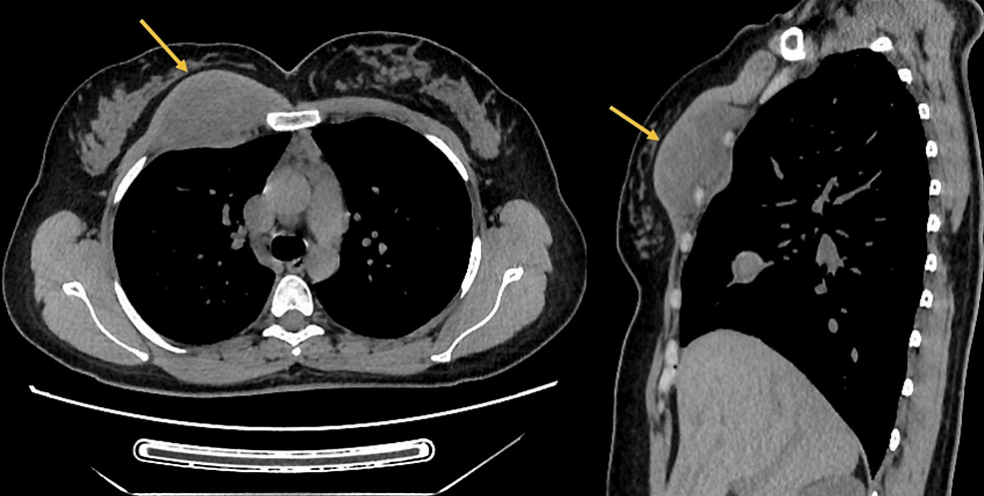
Axial (A) and sagittal (B) sections of the chest CT scan Images showing a right anterior chest wall mass (yellow arrow) infiltrating the parietal pleura
